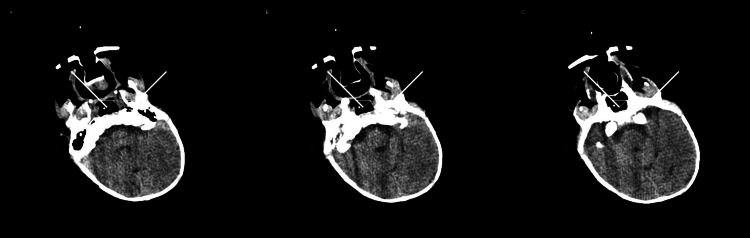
Cranial computed tomography demonstrating craniosynostosis involving the sphenoid and temporal bones bilaterally (arrows)
